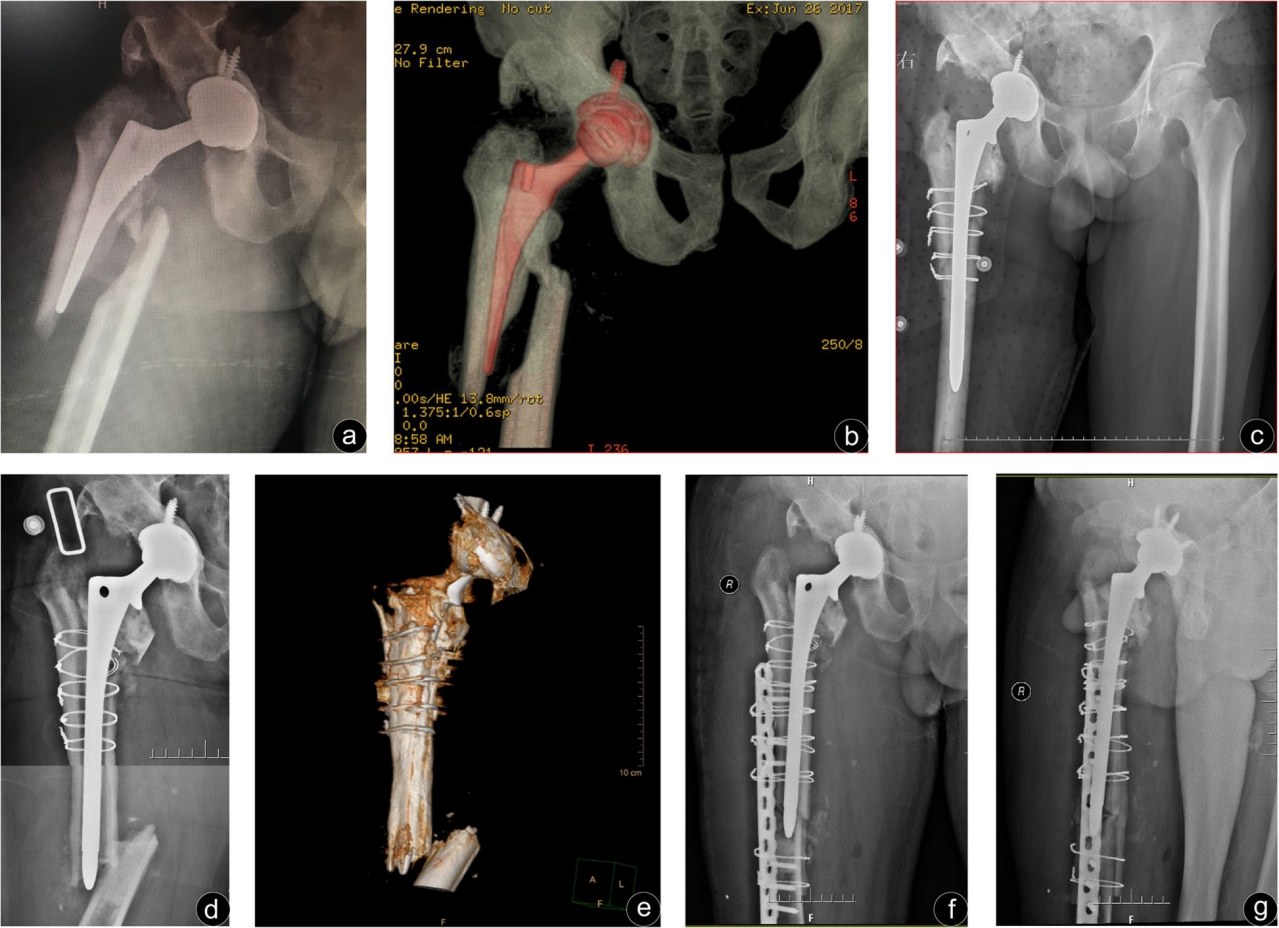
A 53-year-old male who sustained a periprosthetic femur fracture 2 years after primary total hip arthroplasty. Three years after revision, there was another periprosthetic fracture. a, b Preoperative anteroposterior X-ray and three-dimensional reconstruction of the femur showed a spiral fracture. No signs of prosthesis loosening were observed. c The femoral stem was loosened intraoperatively and long-stem revision was used for treatment. d, e The anteroposterior radiographs and three-dimensional reconstruction of the femur showed a short transverse fracture. f, g The anteroposterior radiographs on the 2 weeks after the operation which use locked compression plate Fixation, with allograft strut showed satisfactory fracture reduction and good alignment
